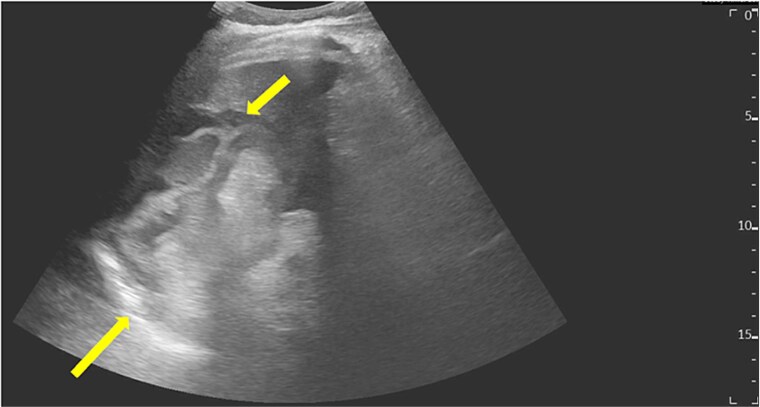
Abdominal ultrasound showing a spleen of average size with preserved contour and echotexture, associated with minimal intraperitoneal free fluid. An ill-defined structure is seen adjacent to the spleen beneath the left hemidiaphragm.
